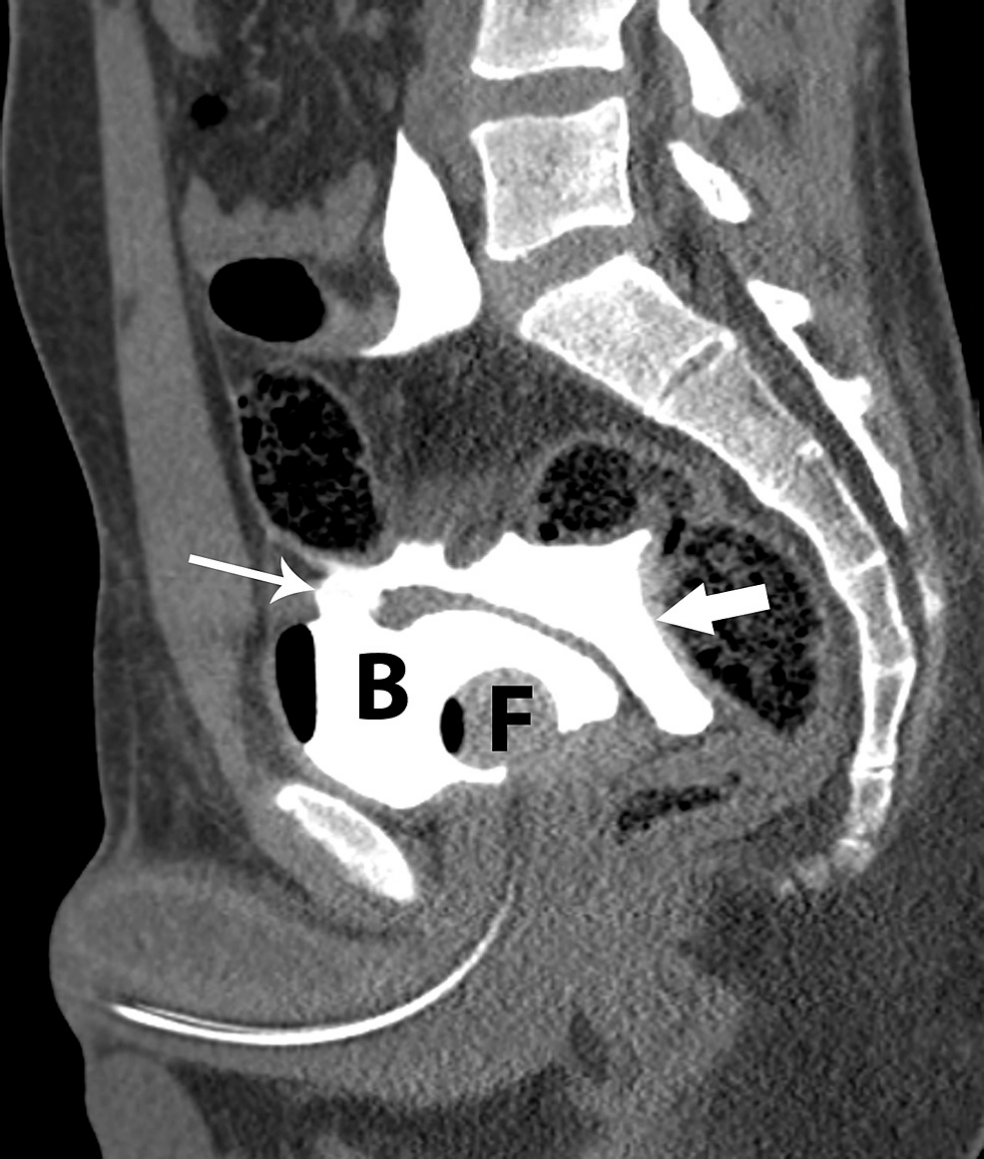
Sagittal reformatted CT image from CT cystogram after injection of contrast material through Foley catheter (F) shows rupture of bladder dome (thin white arrow) with extravasation of dense contrast material (thick white arrow) into the peritoneal space.
